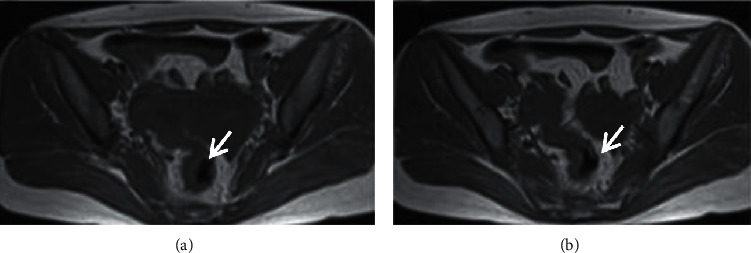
MRI images of FPA patients. (a, b) MRI plain scan images with a layer thickness of 6 mm and an interval of 7 mm, respectively. The fat borders were blurred, and the multiwinding shadows were visible.
